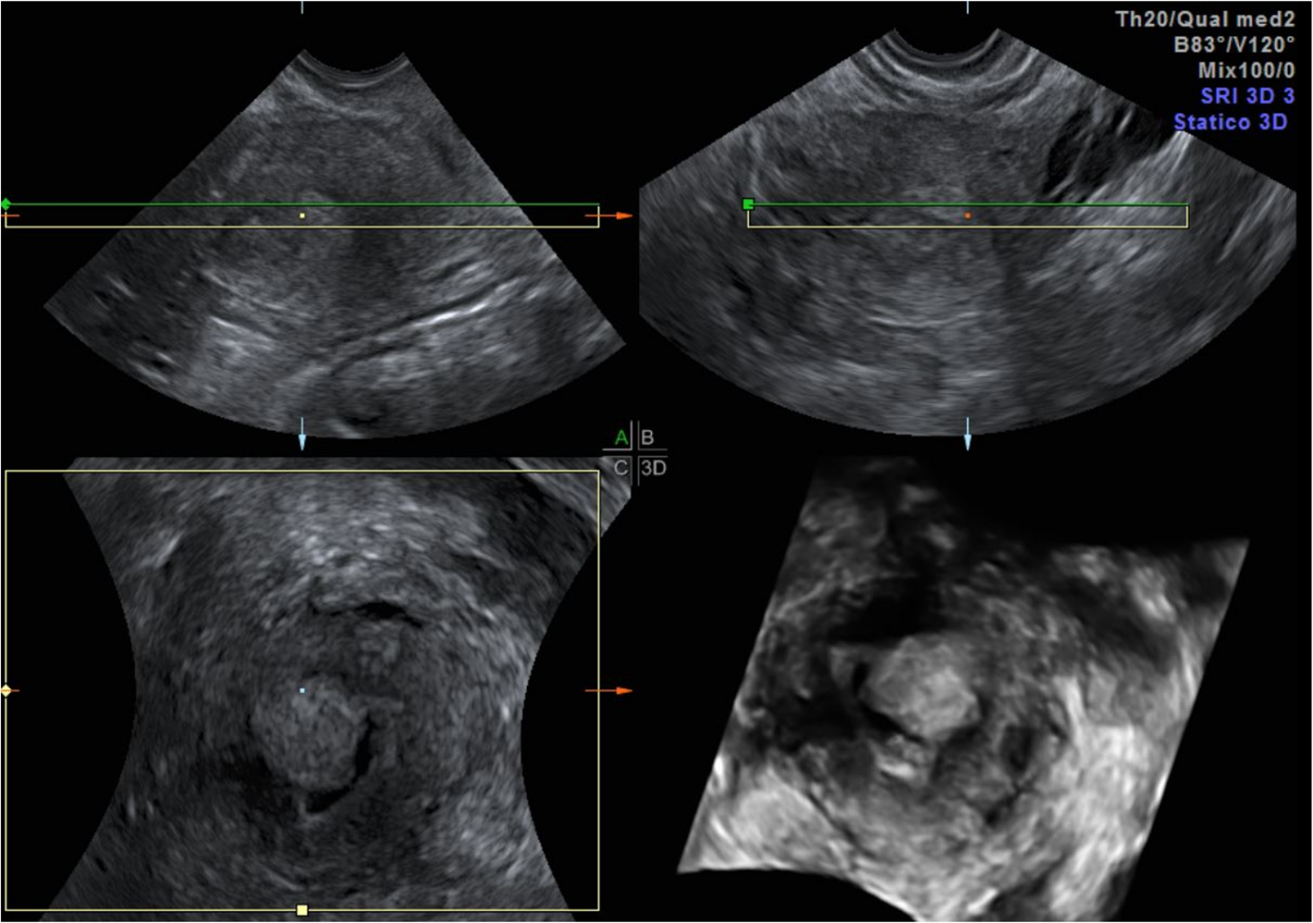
A 52-year-old woman with endometrioid adenocarcinoma (stage IA). Transvaginal ultrasound shows the endometrial cancer invading < 50% of the myometrium
